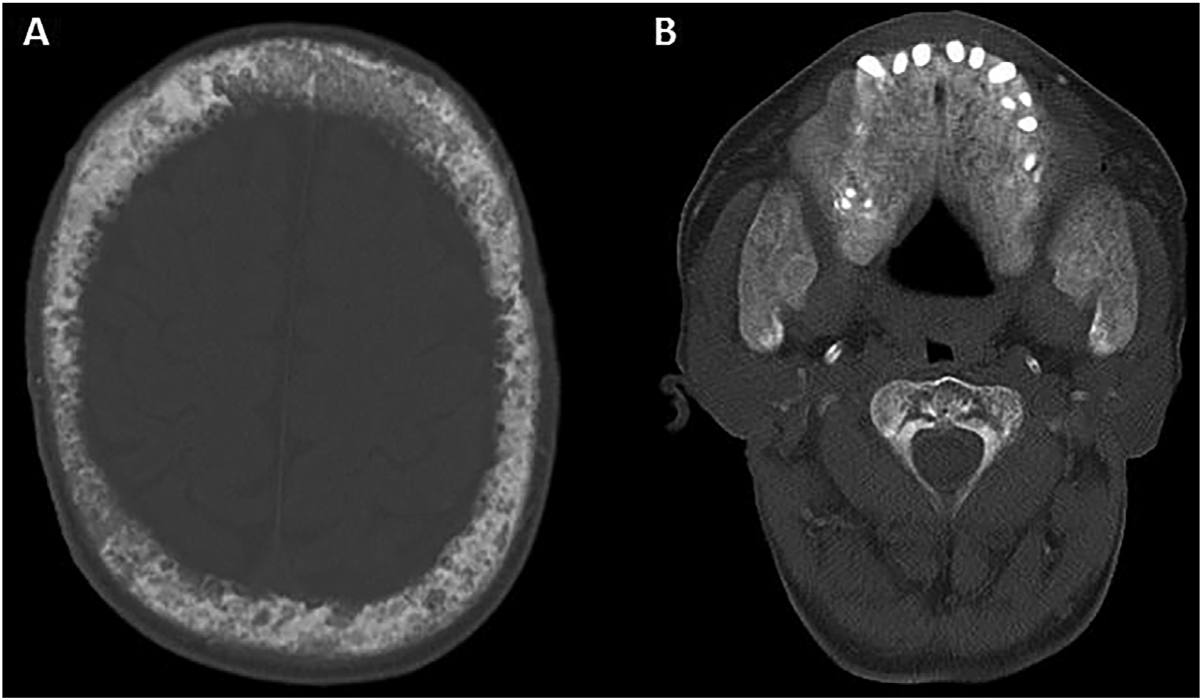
Axial CT of the patient's skull. Thickening was observed in most of the cranial bones, and the maxilla and mandible were profoundly affected. (*A*) There was heterogeneous marked widening of the diploic space of the skull with sclerotic and lytic changes. (*B*) Distinct overgrowths of the ascending rami of mandible with ground glass appearance were seen. The hard palate had been replaced by an enlarged ground glass fibrous structure. CT = computed tomography.
